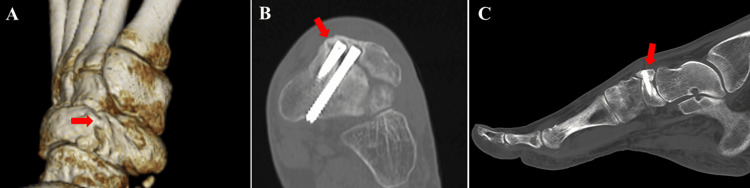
Postoperative computed tomography findings. The partial bone union of the medial side of the fragment was confirmed (red arrows). (A) 3D image, (B) axial view, and (C) sagittal view.
